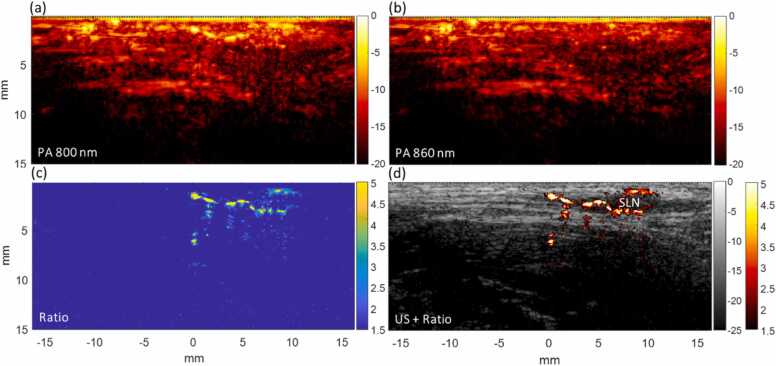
Photoacoustic signal at 800 nm (a) and 860 nm (b) of a superficial inguinal sentinel lymph node (SLN), 30 min after indocyanine green (ICG) administration. Other chromophores are present in both images, while ICG is primarily visible in the 800 nm image. An 800/860 nm ratio image (c) suppresses signal from other chromophores leaving the ICG visible with relatively high ratios. (d) Ratio image overlaid on the corresponding ultrasound image.
